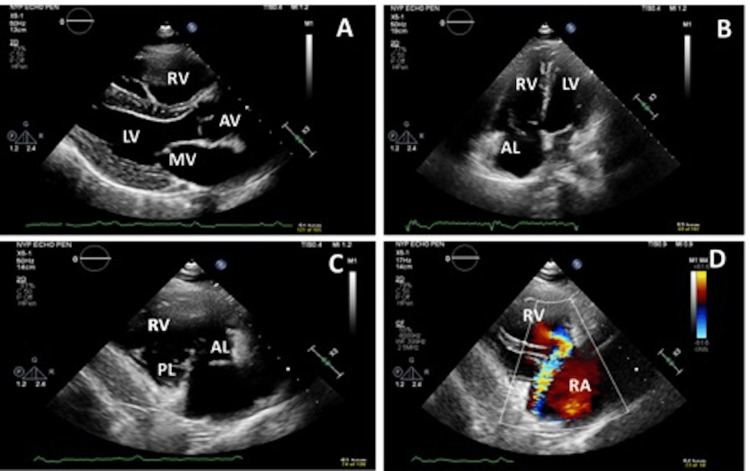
Transthoracic echocardiogram images. A. Parasternal long axis view showing normal mitral valve (MV), aortic valve (AV), and dilated right ventricle (RV); B. Four-chamber view showing dilated right ventricle and prolapse/flail of anterior leaflet of tricuspid valve; C. Right ventricle inflow view showing prolapse/flail of anterior leaflet of tricuspid valve; D. Severe eccentric tricuspid regurgitation
